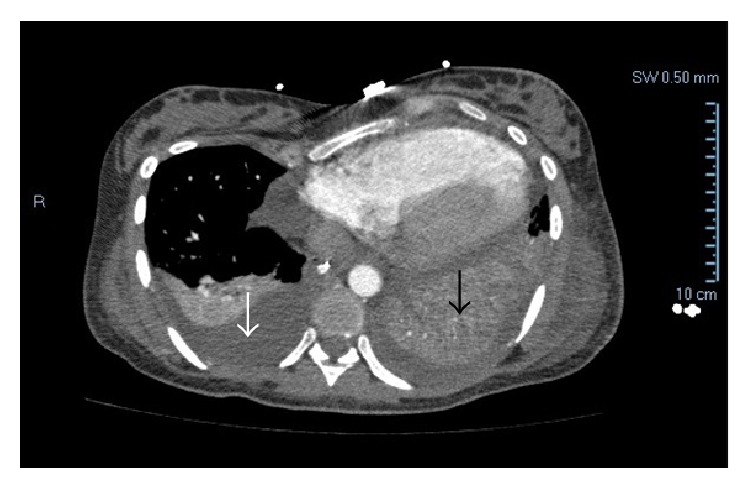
Axial chest computed tomography scan with intravenous contrast revealing right pleural effusion (white arrow) and left pleural effusion with consolidation (black arrow).
